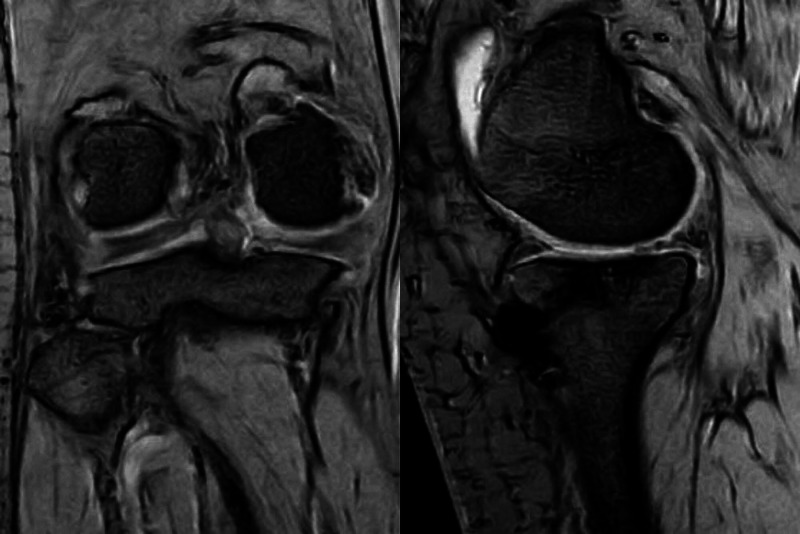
Postoperative MRI (two years later) Medial meniscus posterior root was observed to connect to the insertion site MRI: magnetic resonance imaging
